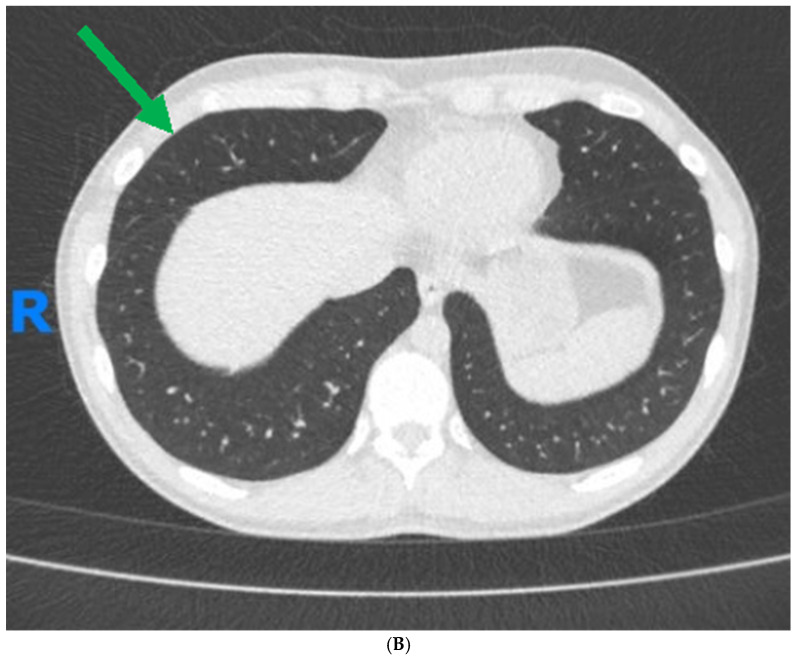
(A)—Chest CT scan revealed subtle opacities in a tree-in-bud pattern in the lower and right middle lobes of the lungs (red arrows), along with minute, non-specific nodules in the lingula and upper lobes. (B)—Follow-up chest CT scan, four months post-treatment initiation, indicated complete resolution of the previously noted nodular opacities (green arrow) and other abnormal findings.
